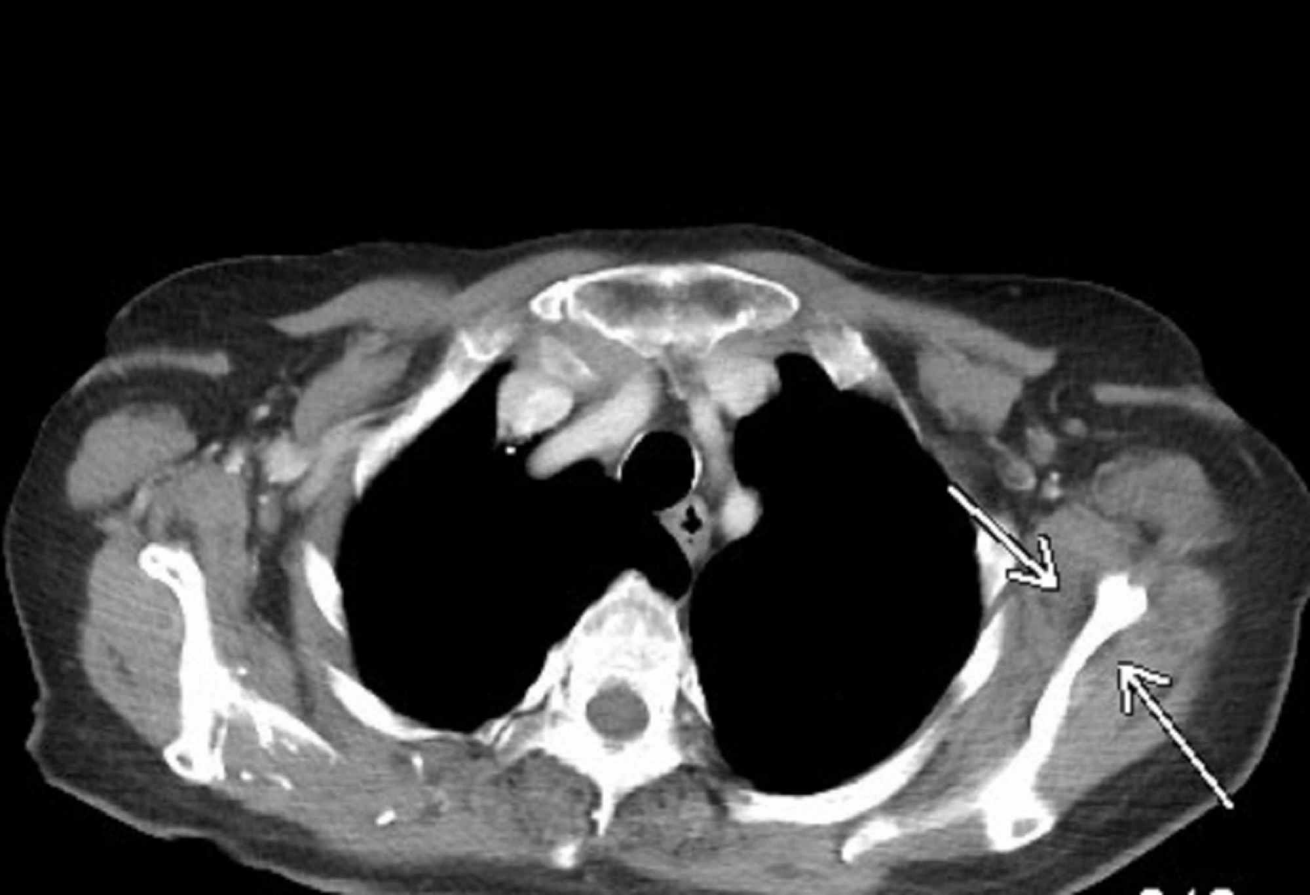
CT chest continues to show complete response of the osseous scapular lesion after being off nivolumab for three years.
